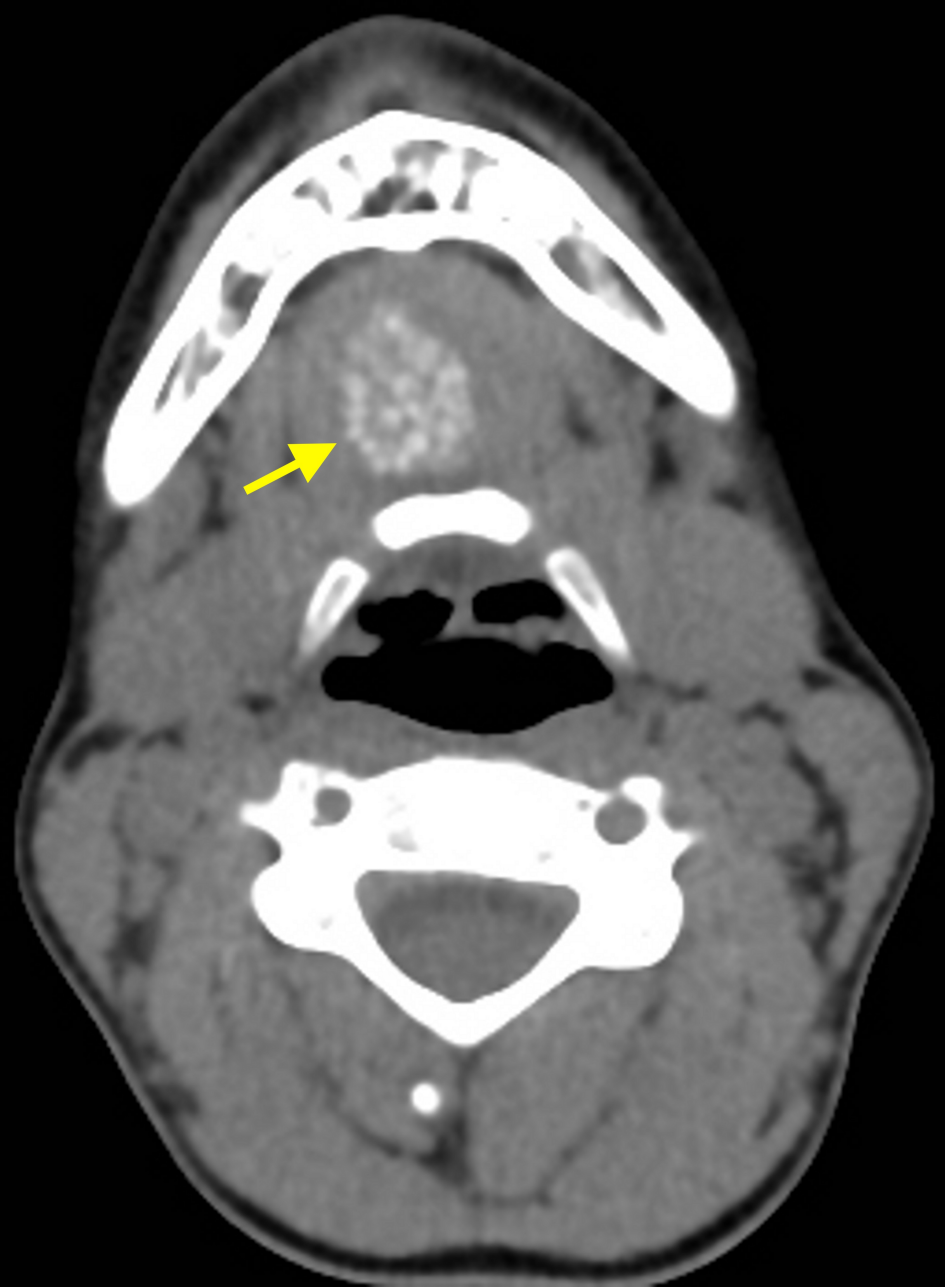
Computed tomography scan The intracystic free corpuscles are calcified (arrow) on non-enhanced computed tomography scan.
